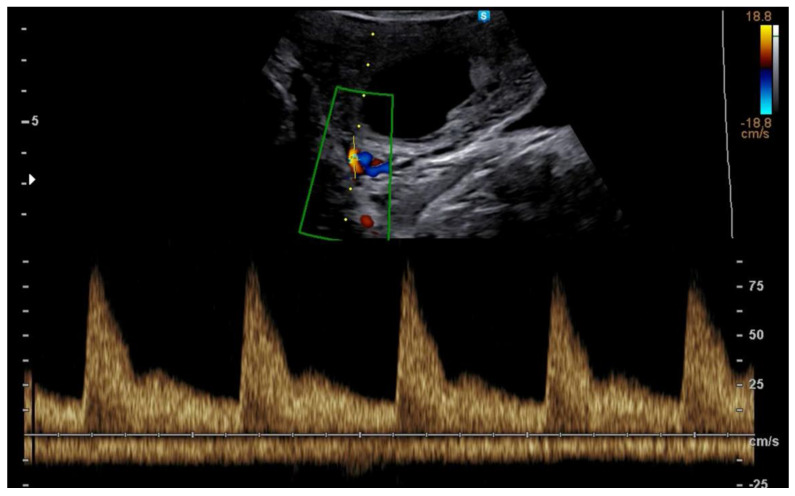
Example of Doppler tracing obtained at 12 weeks from the uterine artery.
